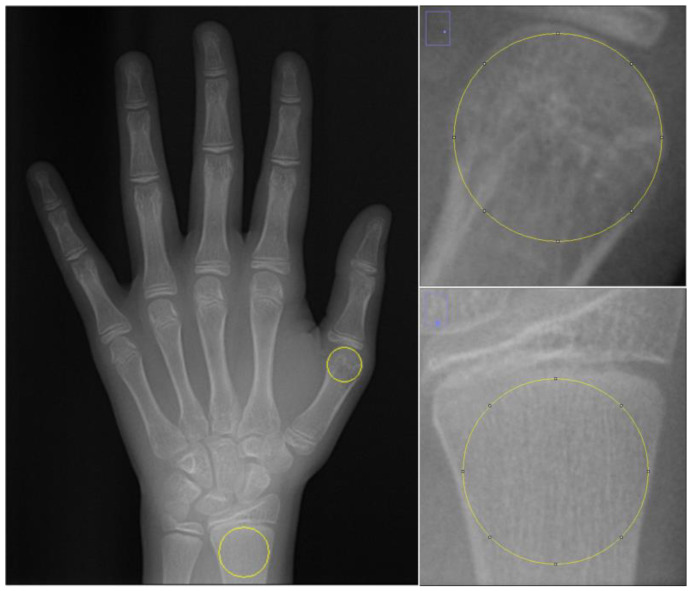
Selection of ROI areas in hand–wrist radiographs.
